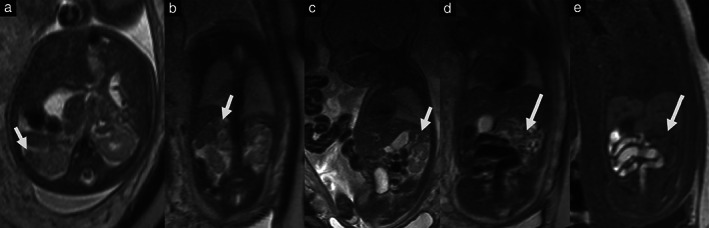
Magnetic resonance images in a fetus with left atrial isomerism at 28 + 6 weeks' gestation, showing polysplenia (short arrow) (a–c) and intestinal malrotation, demonstrated by absence of colonic flexure in left epigastric region (long arrow) (d,e).
